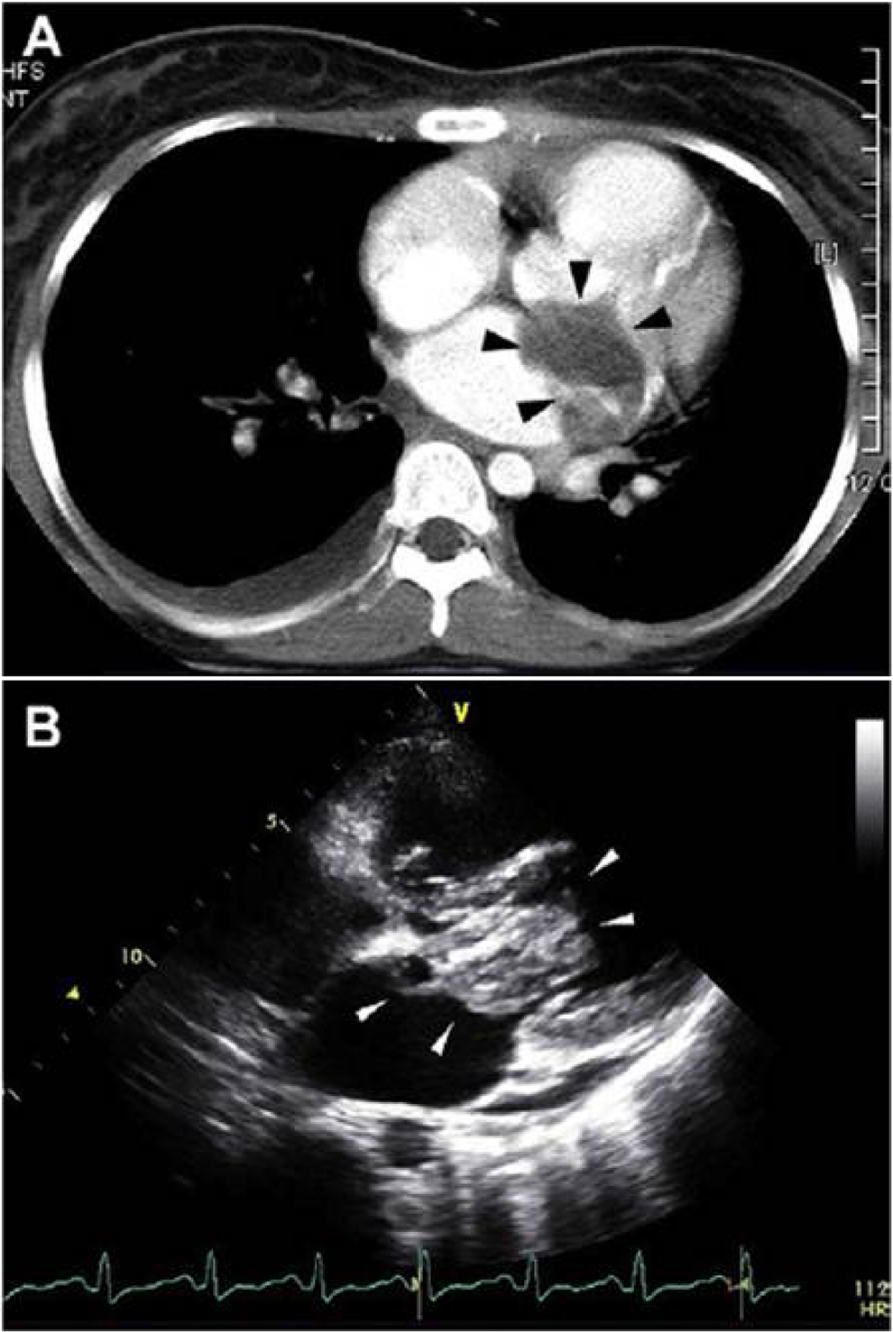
Rhabdomyosarcoma. a. Non-gated contrast CT demonstrating a large mass in the left atrium with heterogenous contrast enhancement (black arrows). b. Transthoracic echocardiogram demonstrates a large mass involving the mitral valve apparatus. Pathology was consistent with a rhabdomyosarcoma. Reproduced with permission from* [25]
